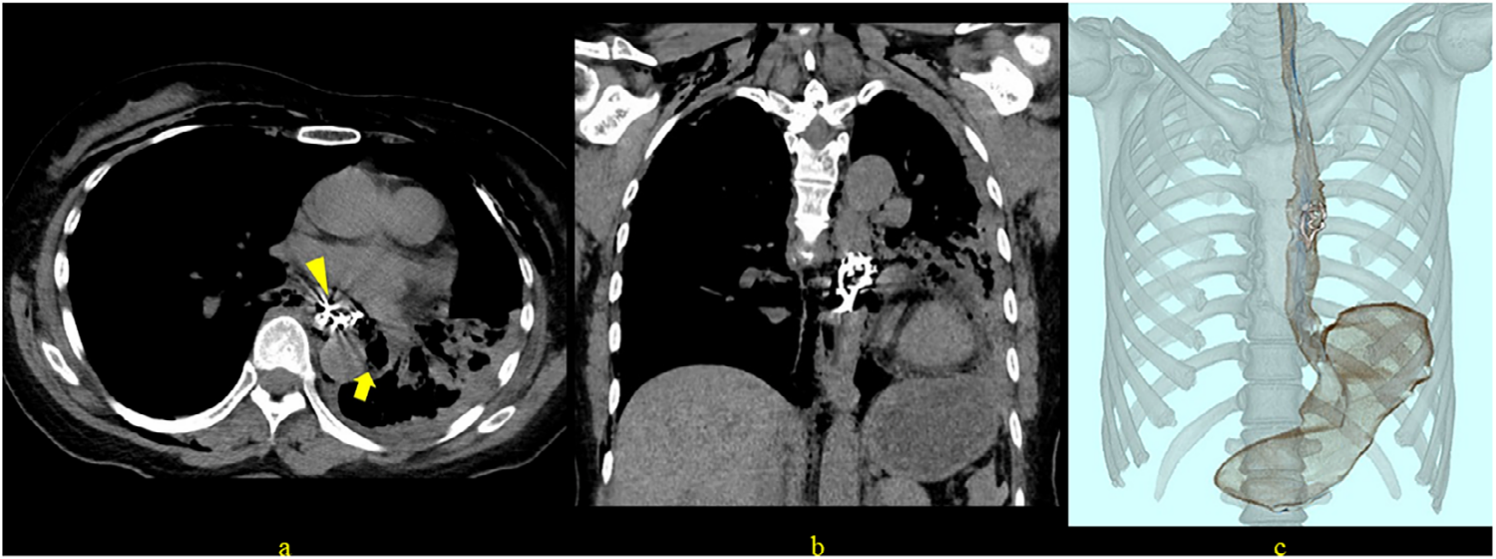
Computed tomography (CT) images at admission. (a) Axial view showing a high‐density structure located in the esophagus (arrowhead) with mediastinal emphysema (arrow). (b) Coronal view revealing the longitudinal extent of the impacted denture. (c) 3D reconstruction image clarifying the shape of the bridge‐type denture and its positional relationship with the surrounding structures.
